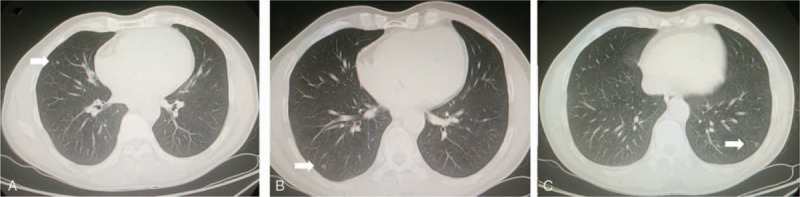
The CT on admission revealed synchronous triple pulmonary nodule measuring 5 to 7 mm in diameter located separately in different lobes.
